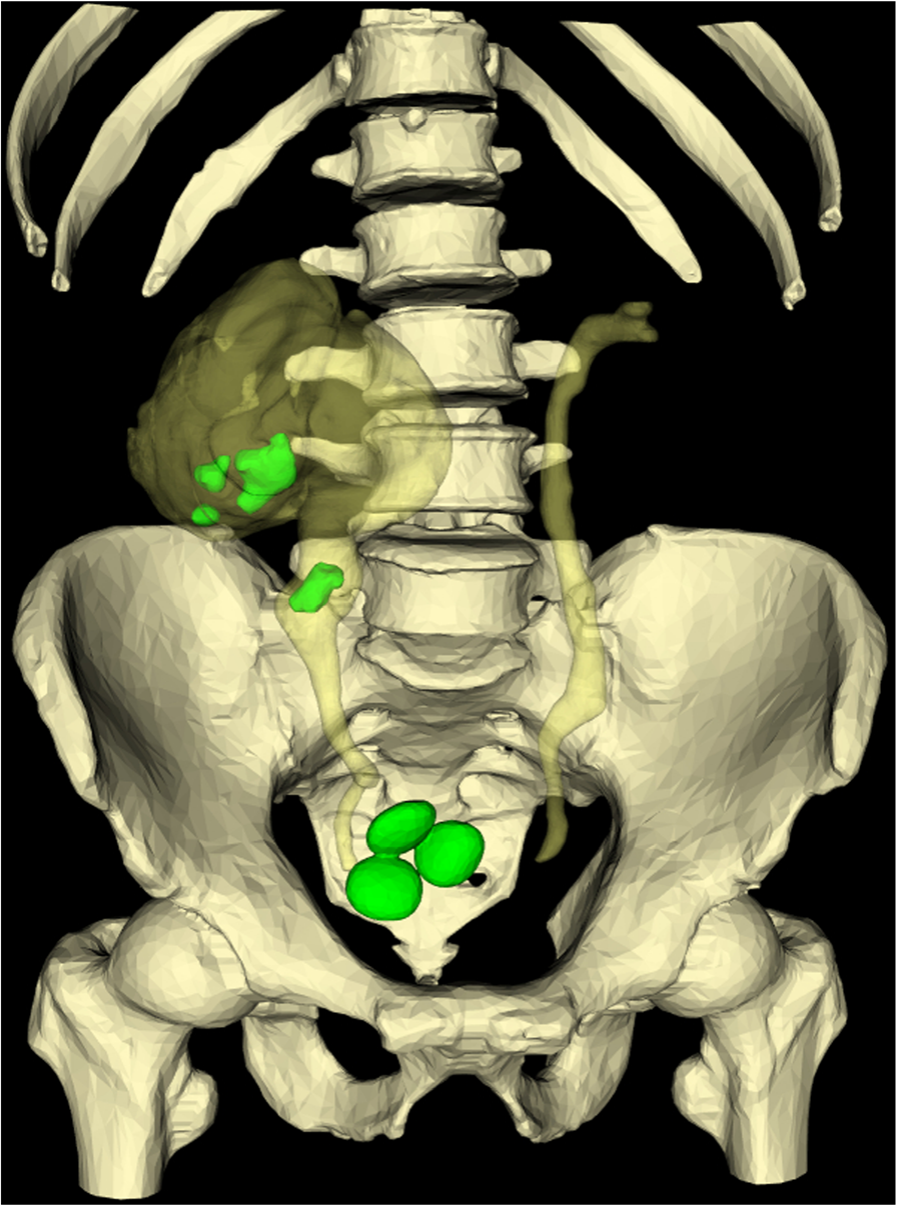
Three-dimensional reconstruction CT image showing the multiple urinary tract calculi and the morphology of the urinary tract
